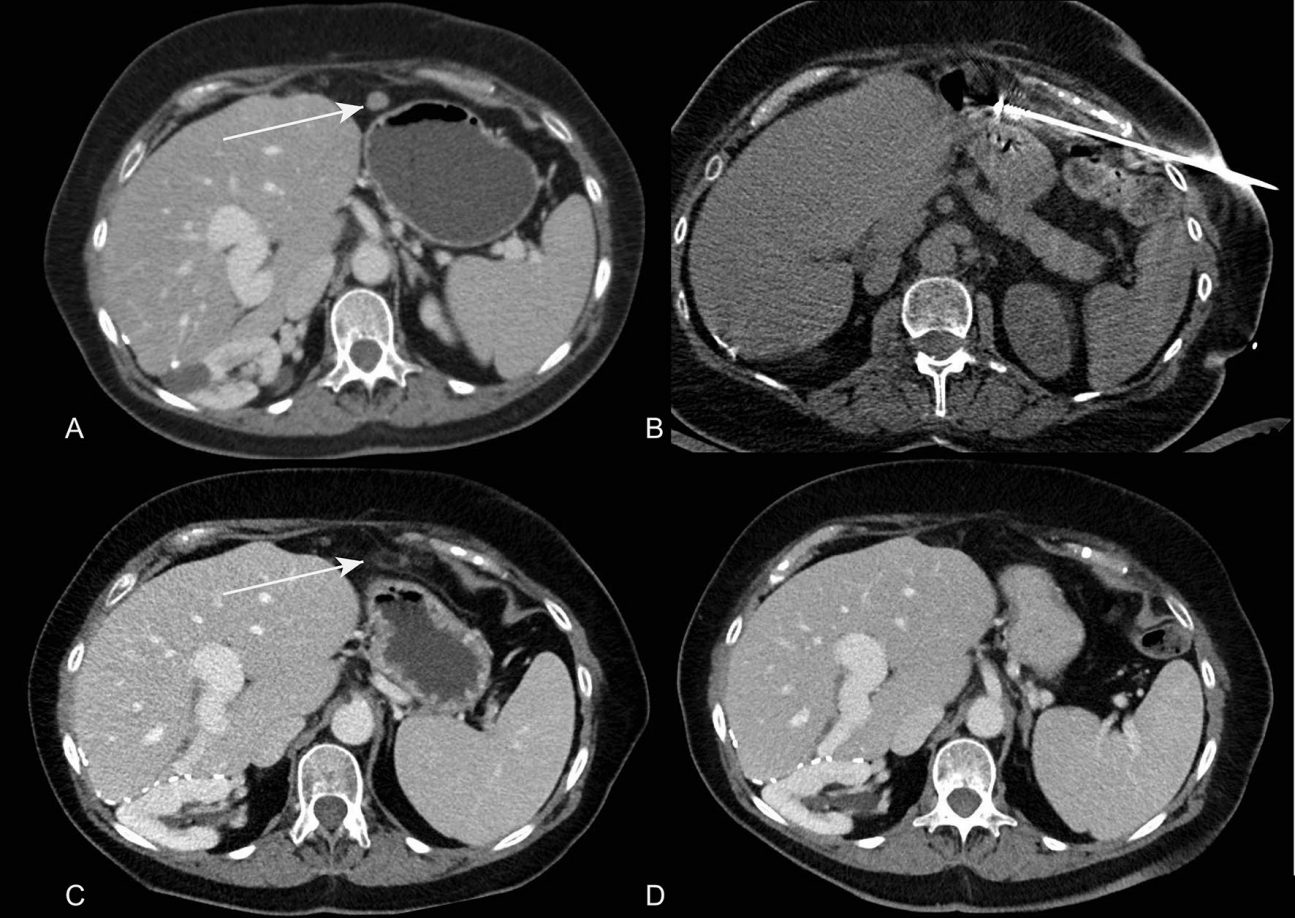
A 67-year-old female patient presents with a peritoneal metastases of granulosa cell carcinoma in the epigastric region of 13 mm (A). Cryoablation with one needle with hydrodissection for stomach proximity (B). Follow-up CT shows complete ablation after one month (C) and persistent local control after 27 months (D)
